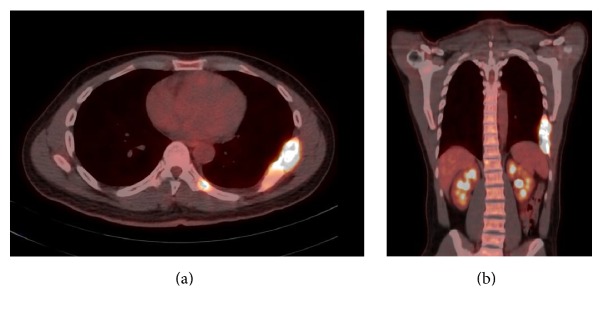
(a) Axial PET-CT demonstrating avid FDG uptake in the destructive lesions involving the 7th, 8th, and 9th ribs. Also a notable focus of FDG uptake is seen left of the T12 vertebra. (b) Coronal PET-CT demonstrating avid FDG uptake in the destructive lesion involving the 7th, 8th, and 9th ribs.
